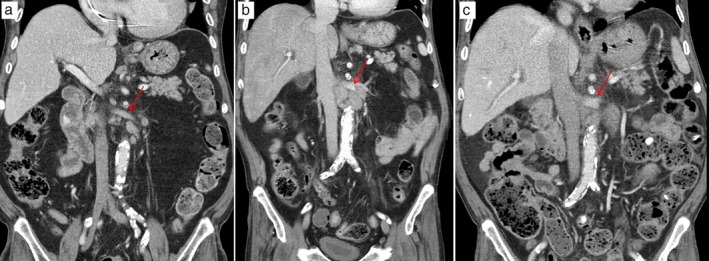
Abdominal computed tomographic (CT) scans. (a) The abdominal CT scan showed multiple small lymph nodes at the gastric cardia, on the left gastric artery, and along the paraaortic lymphatic chains. (b) After three months, enlargement of lymph nodes could be seen, especially para‐aortic lymph nodes at the level of the left renal vein. (c). After one month of crizotinib treatment, para‐aortic lymph nodes were markedly regressed. *arrows, left renal vein.
